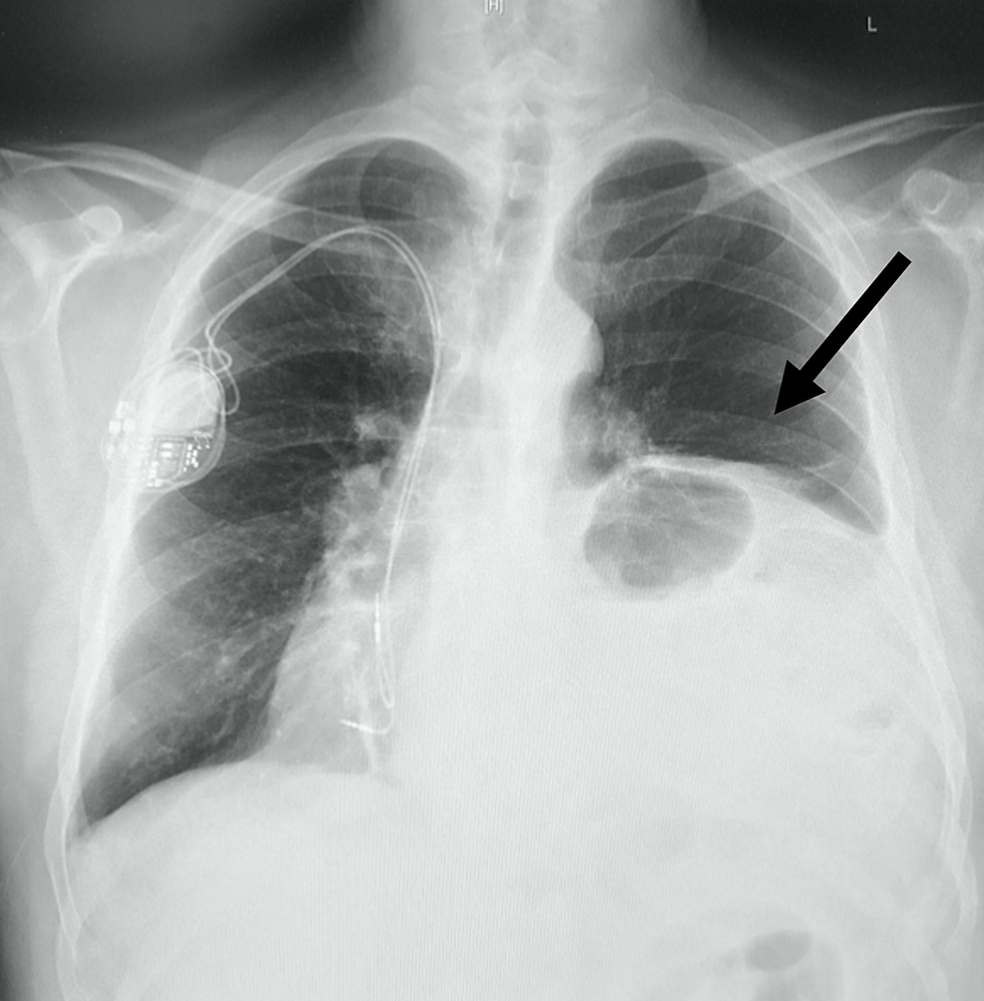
Anterior-Posterior Chest Radiograph This anterior-posterior chest radiograph demonstrates a marked rightward mediastinal shift and asymmetric elevation of the left hemidiaphragm with presumed voluminous hiatal hernia (arrow denotes the herniated abdominal contents in the left thoracic cavity), with pacemaker placement in the right chest wall.
